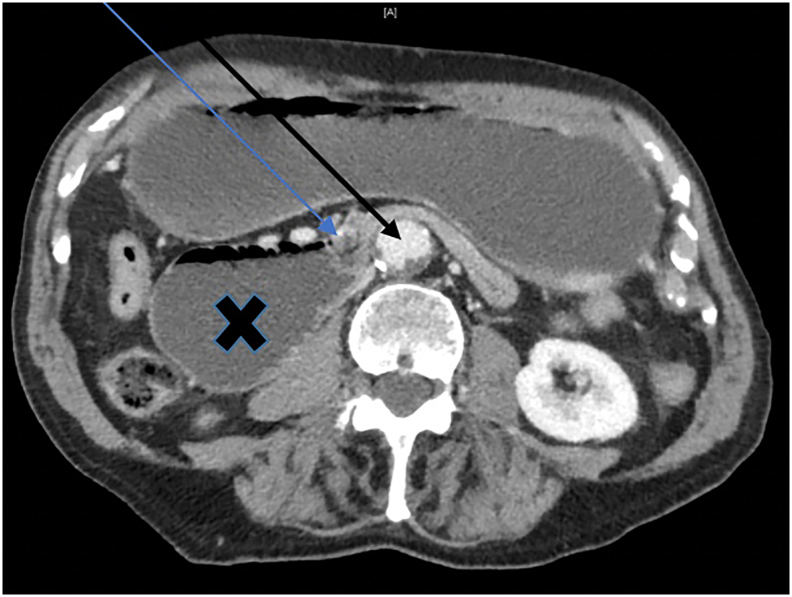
Computed tomography Axial view demonstrating extensive dilatation of proximal duodenum marked by X, between SMA and AAA marked by blue and black arrow respectively.
